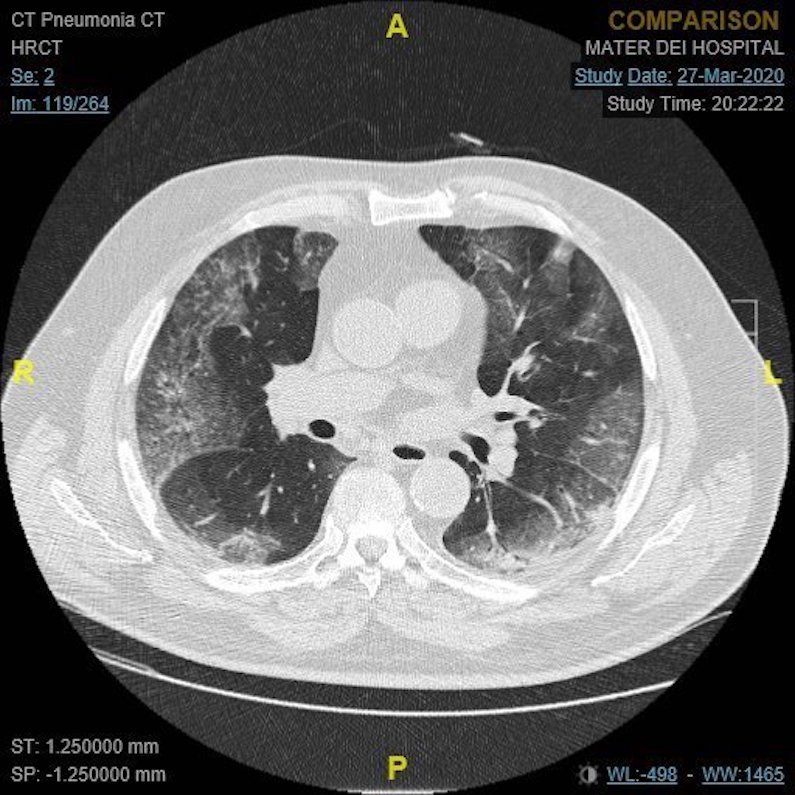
A non-contrast CT showing extensive ground-glass changes in both lung lobes with predominant peripheral distribution, compatible with moderate-severe bilateral COVID-19 pneumonia.
